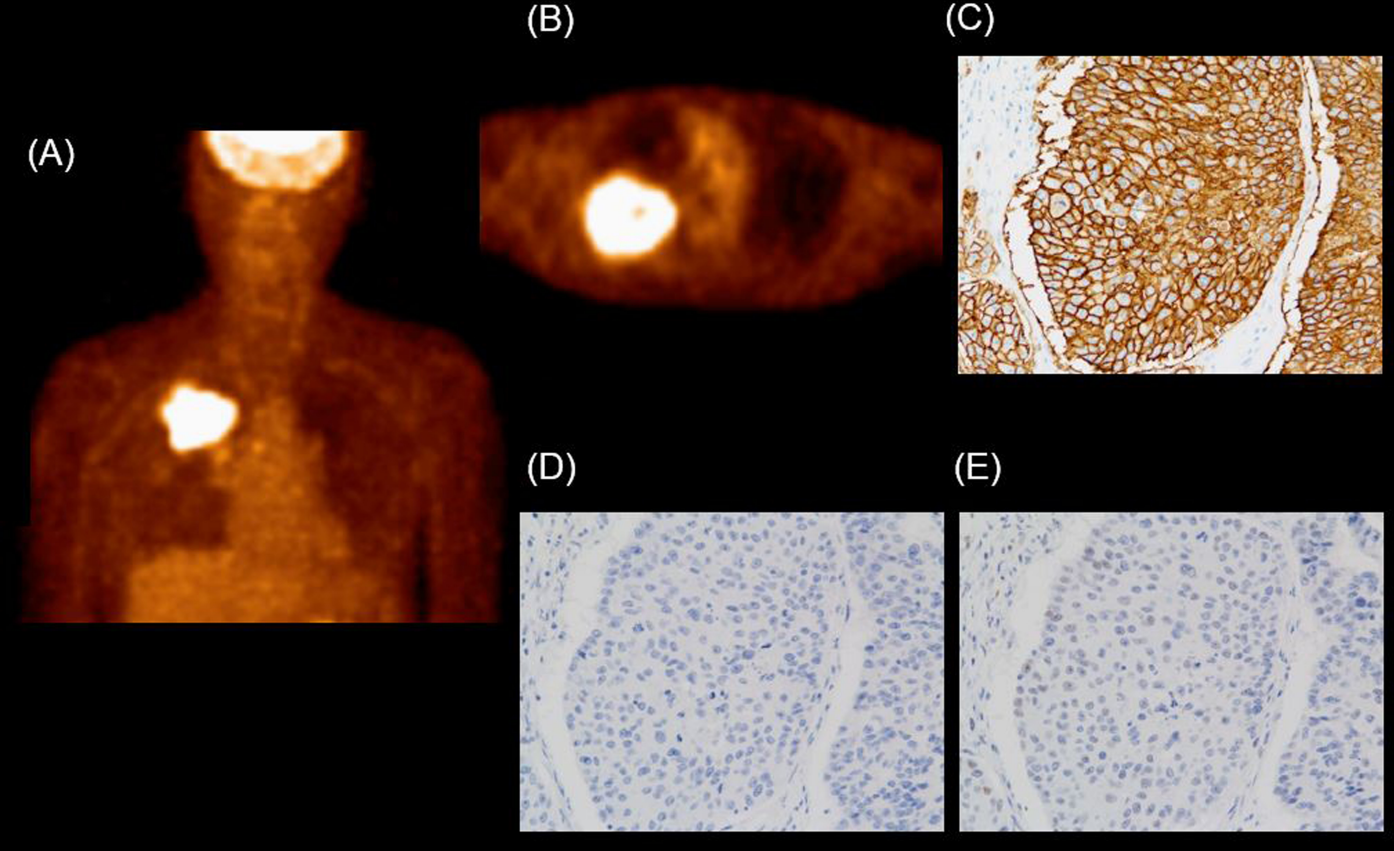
Example of NSCLC (adenocarcinoma; pT2N0M0 stage IIA) in an 81-yr-old man High ^18^F-FDG uptake is observed in the right upper lung field. (A) MIP image. (B) Axial PET image. SUVmean: 5.39, SUVmax: 8.30, MTV: 110.3, and TLG: 595.31. (C) The Glut1 score was 210. (D) The pStat1 expression score was 1. (E) The pStat3 expression score was 30.
